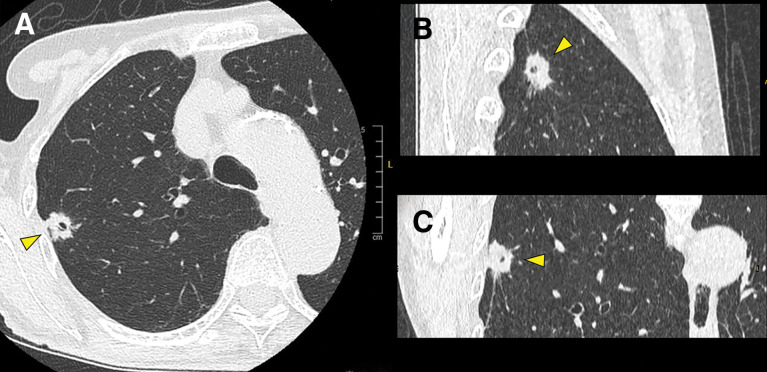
Chest CT. A 1.6-cm subpleural nodule with irregular margins, spiculation, and an internal cavity (yellow arrowheads) is visible in the right upper lobe. (A) Axial view, (B) sagittal view, and (C) coronal view.
